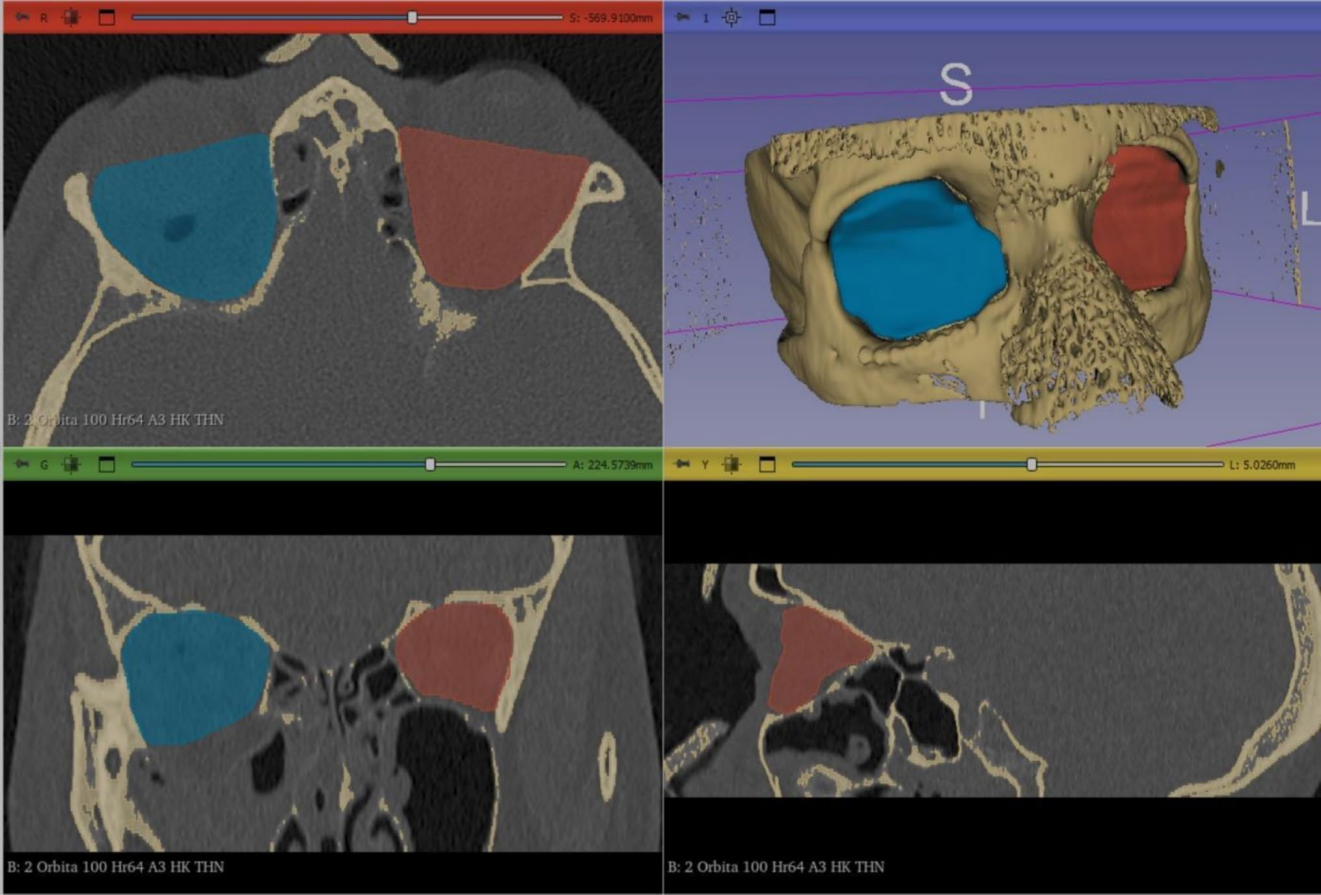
Postoperative CT scan of the midface in axial (A), coronal (C), sagittal (D) and 3D reconstruction (B). Segmented volumes of the fractured and reconstructed (blue) and intact orbit (red) were generated using 3D-Slicer software
